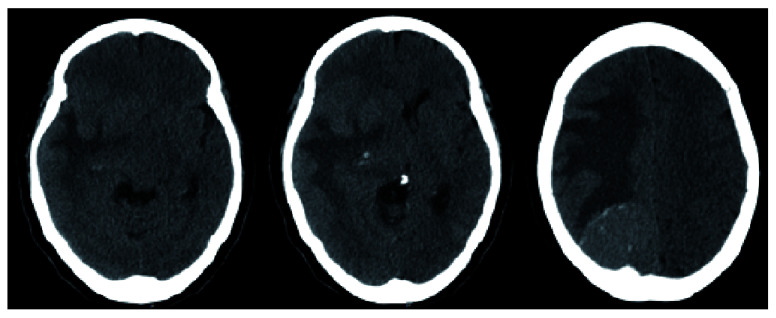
CT head (axial view) demonstrating a calcific right parietal lesion with vasogenic edema as well as a periventricular lesion with associated edema causing 12mm of midline shift.
